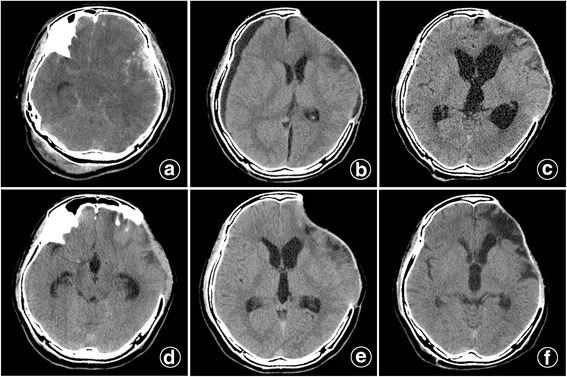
a A acute subdural hematoma in the left frontotemporal. b A repeated CT two months after the initial surgery. c A progressive hydrocephalus three months later. d, e Three days after placing the patient in a Trendelenburg position and giving sufficient hydration, the patient had a SSFS but no obvious midline shift. f Five years later, hydrocephalus improved itself without cranioplasty or ventriculoperitoneal shunt
